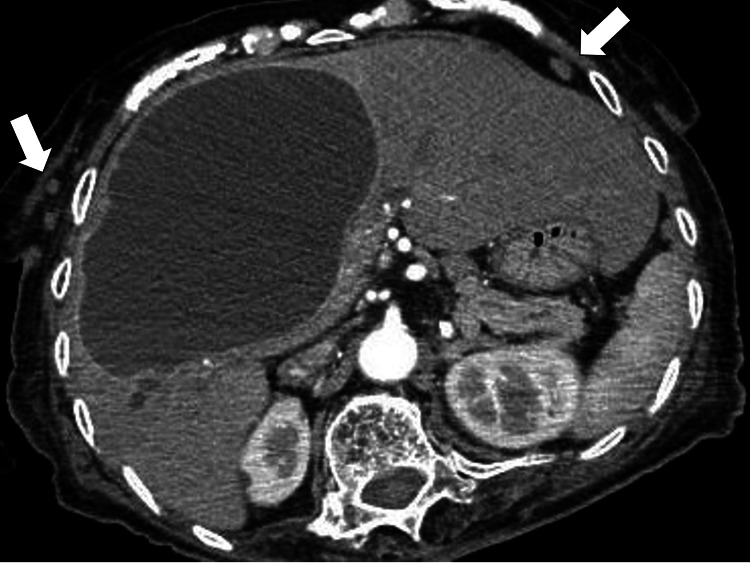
Contrast-enhanced computed tomography showing multiple masses on the chest wall (white arrows) and no mosaic density changes in the intrahepatic cyst
